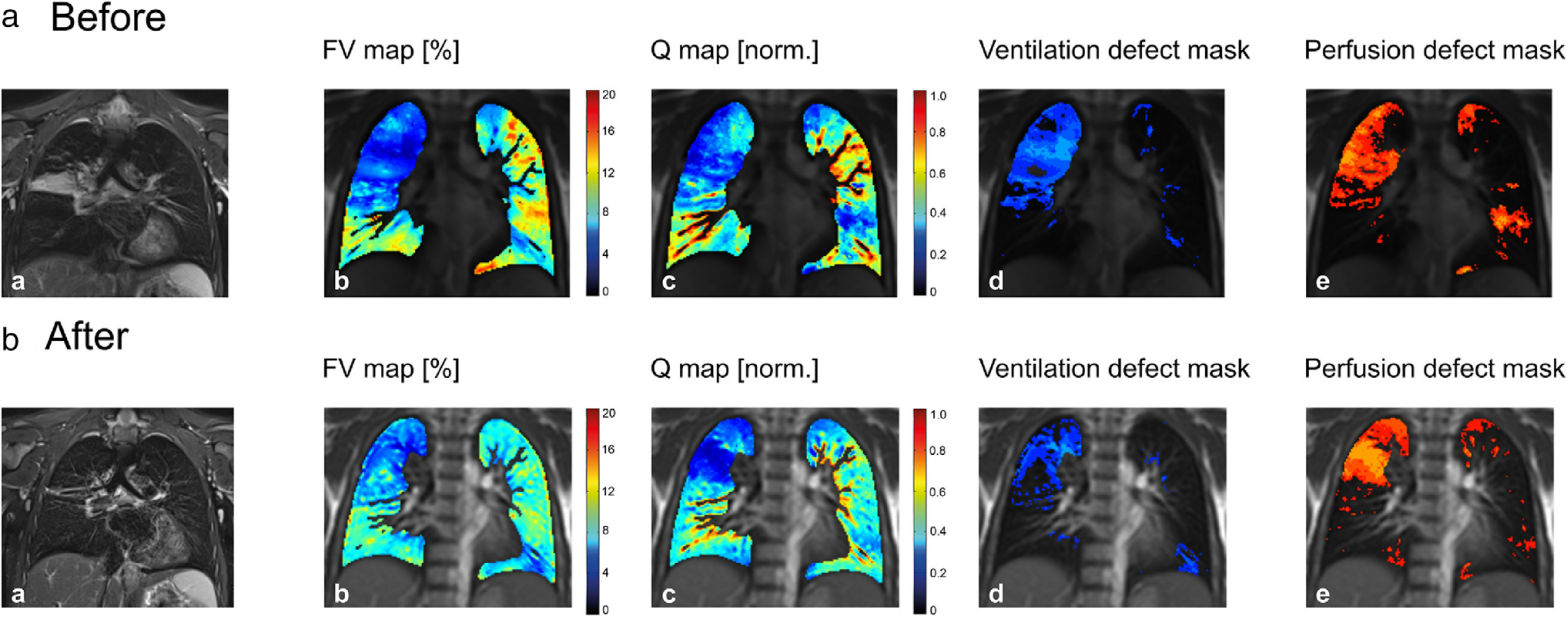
Comparison of pulmonary MRI before and after triple combination therapy in an 11‐year‐old patient with cystic fibrosis. From left to right: morphological (PROPELLER) images, fractional ventilation (FV), perfusion (Q) and ventilation and perfusion defect masks derived from dynamic 2D bSSFP‐based matrix pencil imaging, respectively before (a) and after (b) the therapy showed partial resolution of atelectasis in the right upper lobe and reduced bronchial wall thickening on morphological imaging, and corresponding improvement of ventilation and reduced ventilation‐perfusion mismatch. Reprinted with permission from Reference 128. bSSFP = balanced steady‐state free precession; PROPELLER = Periodically Rotated Overlapping ParallEL Lines with Enhanced Reconstruction.
